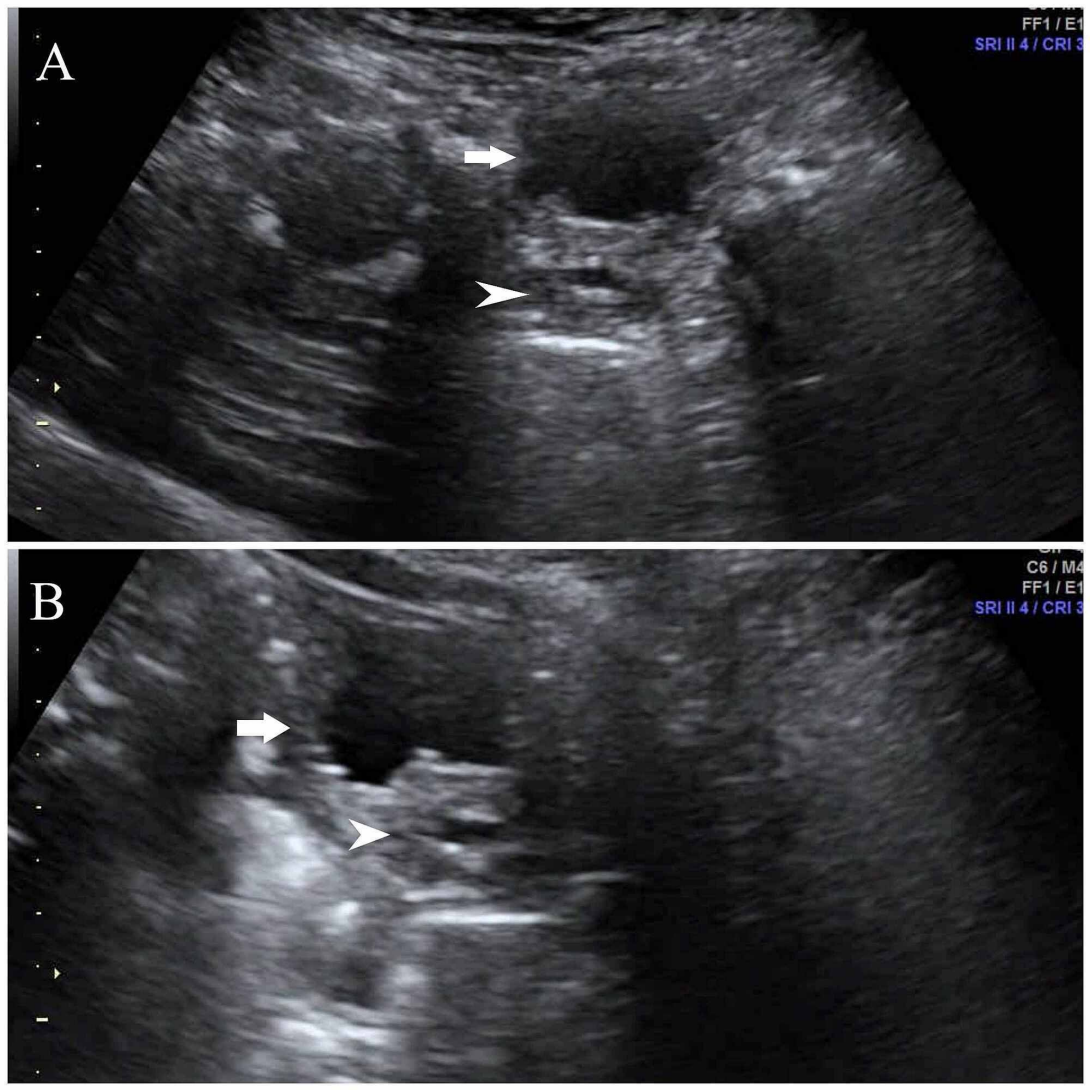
Repeat ultrasound of urinary bladder and prostate after drainage of pus A – Axial image, arrow shows contracted urinary bladder, and the arrowhead shows the tiny central hypoechoic focus of size 6 x 5 mm suggesting residual component. B – Sagittal image, arrow shows contracted urinary bladder, and the arrowhead shows the tiny central hypoechoic focuses suggesting residual component.
